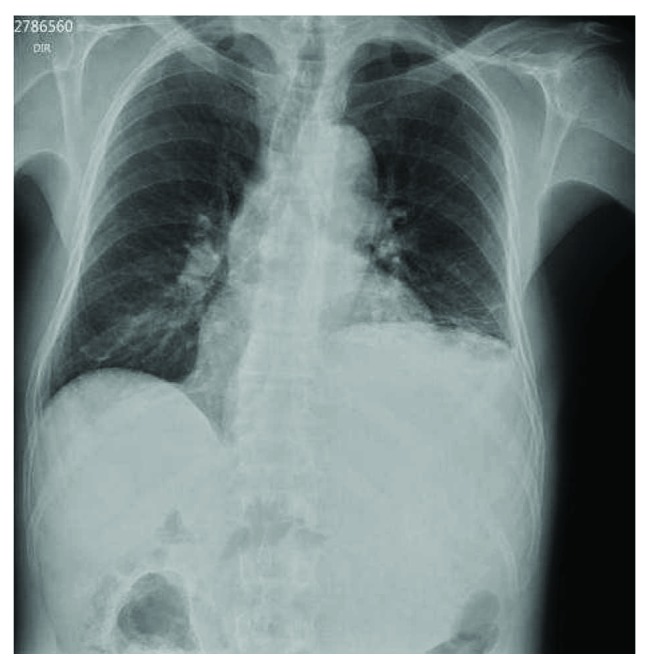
Acute abdominal X-ray showing the chest with elevated left hemidiaphragm.
